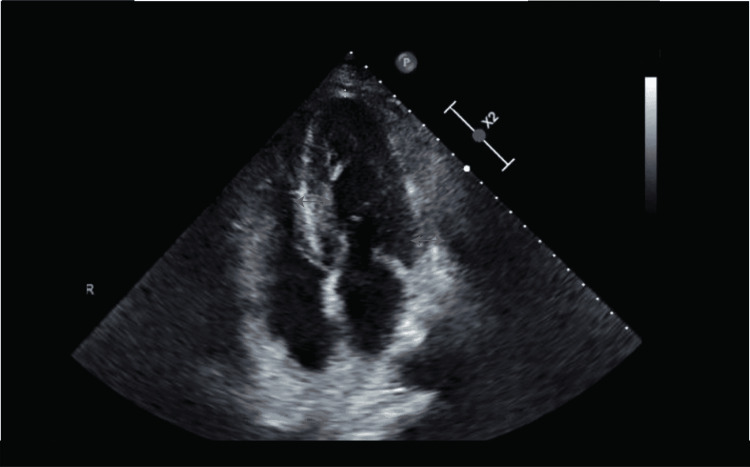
Transthoracic echocardiogram Apical 4 chamber view demonstrating increased septal wall thickness (red arrow), and lateral left ventricle wall (green arrow), with apical sparing (yellow arrow).
